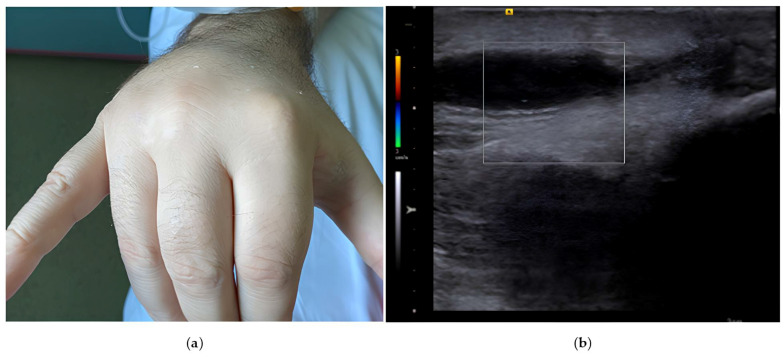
(a) Swelling in the dorsal region of the right hand, with flexion deformities of fingers II–IV and extension of the fifth finger and thumb. (b) Ultrasound examination of the hand showing an anechoic area with no Doppler signal adjacent to the extensor tendons, suggestive of tenosynovitis.
